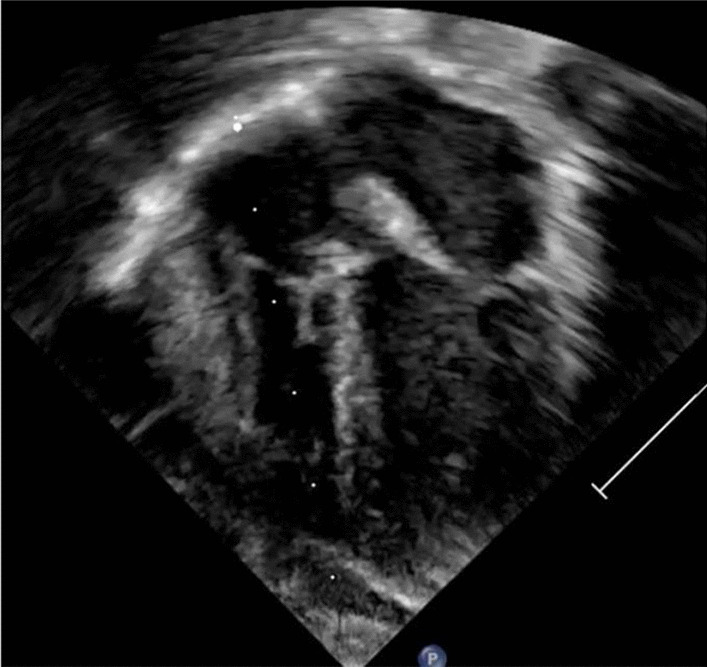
Postnatal two-dimensional echocardiogram. Legend: Four-chamber view showing a mass arising from the right ventricular wall
